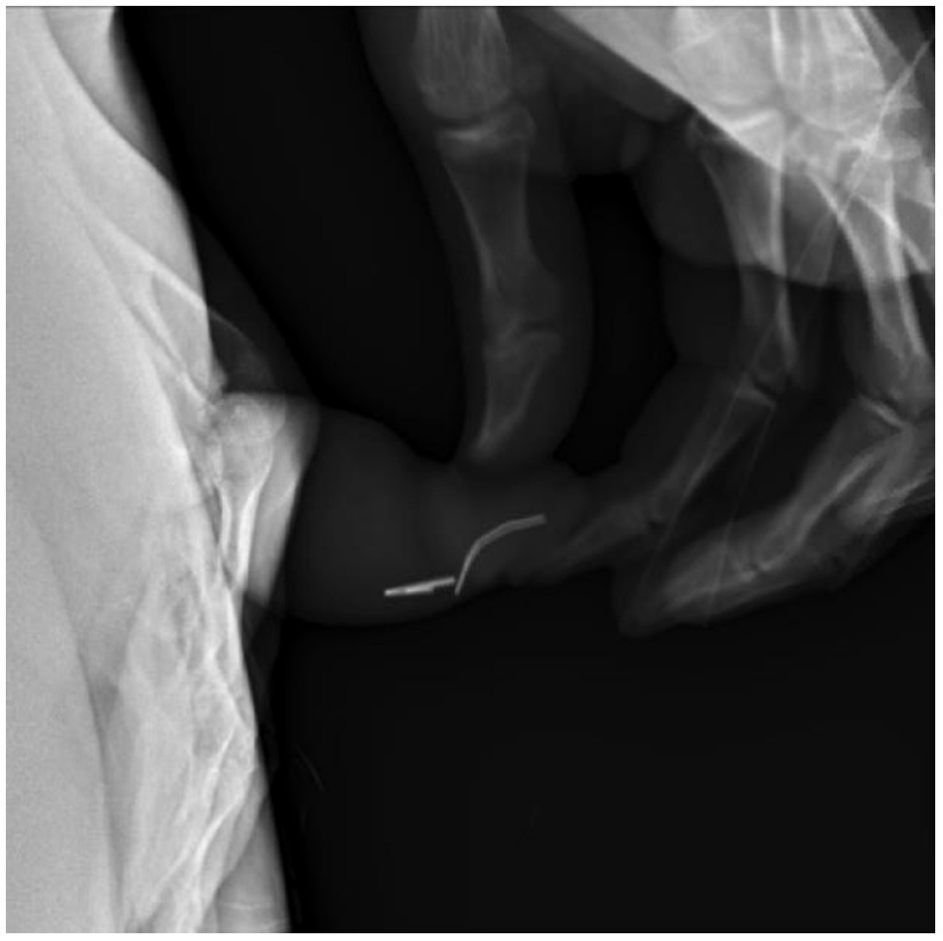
Lateral view pelvic X-ray identifies staples in distal penile urethra, which requires endoscopic removal.
